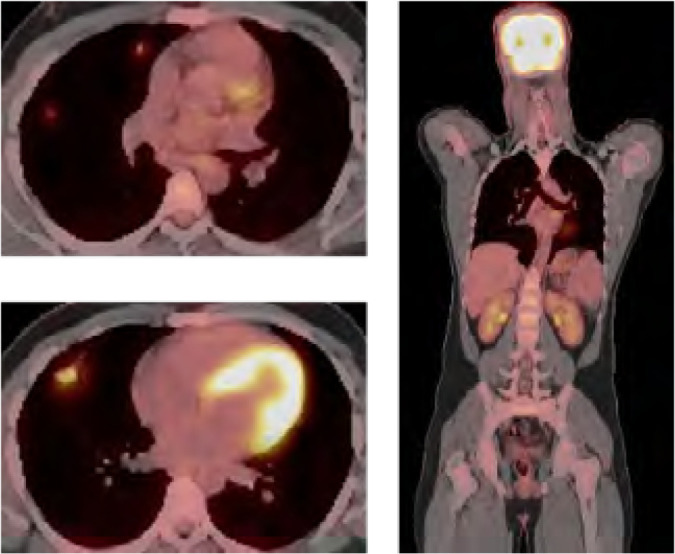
PET-CT axial and coronal images show hypermetabolic parenchymal lung nodules (SUVmax: 2,1–8,1). FDG distribution in other parts of the body is within normal limits.
